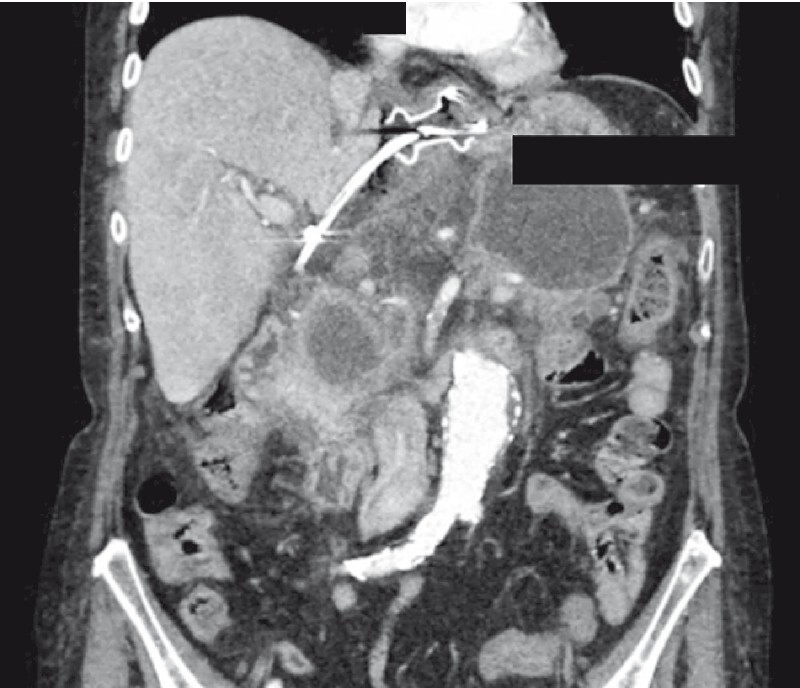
Computed tomography scan showed the lumen-apposing metal stent and the pigtail stent close to the hepatic hilus.
